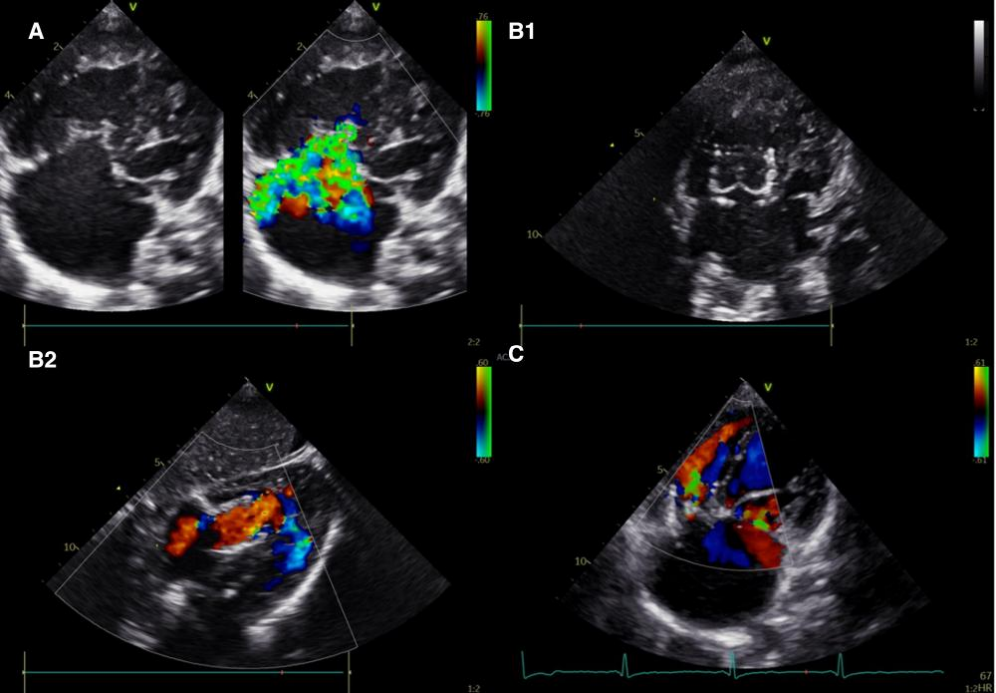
Evolutive functional changes in tricuspid valve (Case 2). (*A*) Transthoracic echocardiogram showing severe tricuspid regurgitation pre-surgery. (*B1*, *B2*) Transthoracic echocardiogram immediately after implant of the bioprosthesis. (*C*) Transthoracic echocardiogram 30 months after the implant with severe tricuspid regurgitation.
